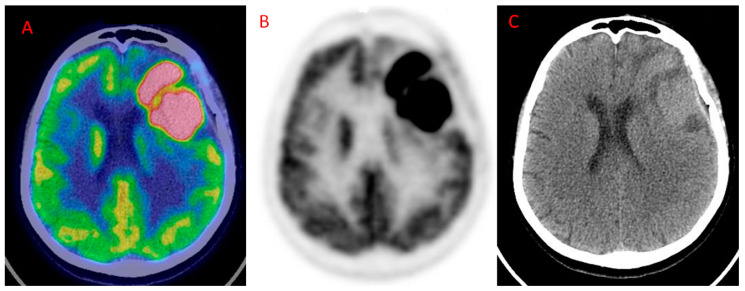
Primary Central Nervous System Lymphoma (PCNSL) in the left frontal cortex showing high ^18^F-FDG uptake. (A) Axial fused ^18^F-FDG PET/ CT; (B) ^18^F-FDG PET; (C): CT. (Courtesy of Dr. Cistaro).
